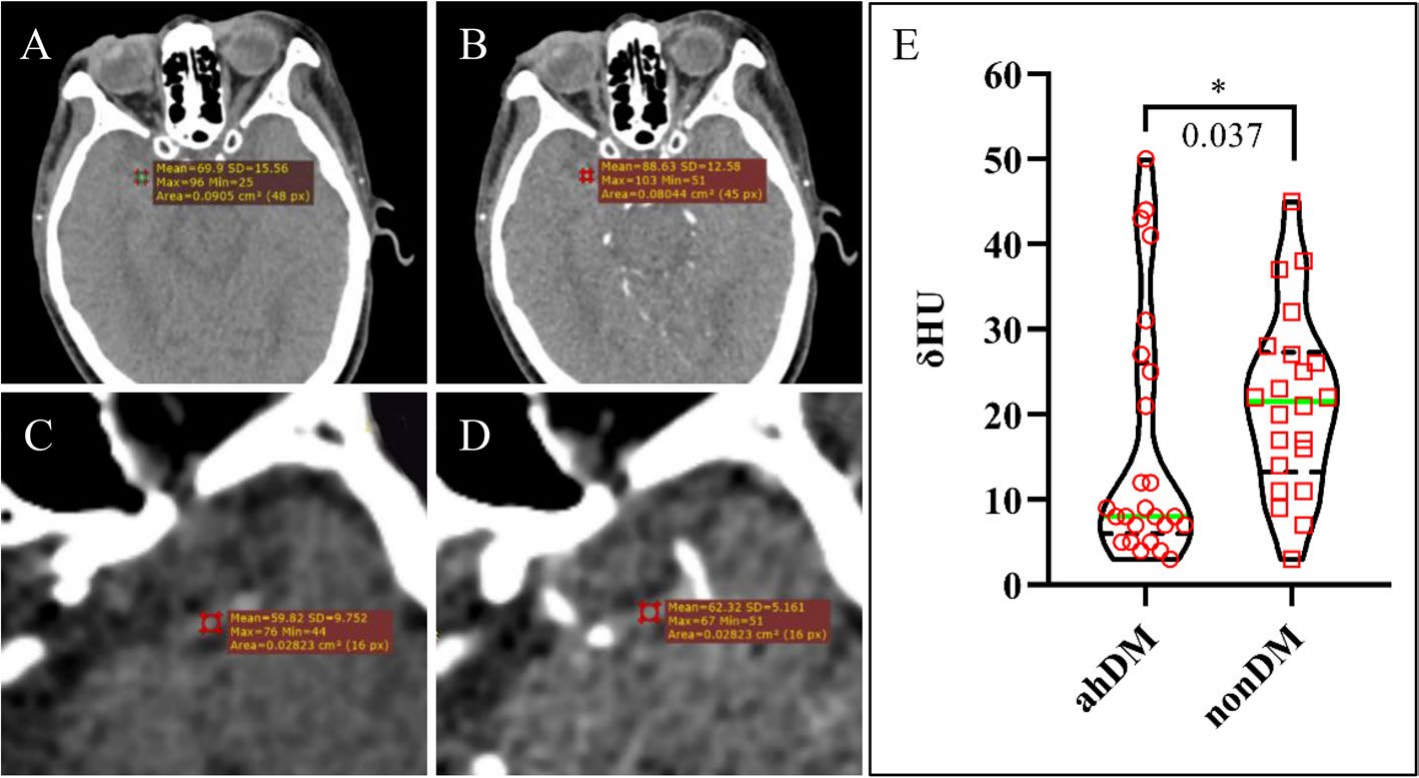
Clot perviousness measurement. Clot permeability was assessed using CT and CTA. Acquired non-contrast CT (A) and CTA (B) images of a representative patient without DM, and digitally zoomed (C, D) representative images of a patient with ahDM depict an occlusive clot in the M1 segment. Regions of interest of the clot assessed on non-contrast CT (A, C) and CTA (B, D). E δHU was significantly lower in patients with ahDM than in patients without DM. CT, computed tomography; CTA, computed tomography angiography; ahDM, admission hyperglycemia diabetes mellitus; δHU, Absolute thrombus perviousness; M1, first segment of the middle cerebral artery
